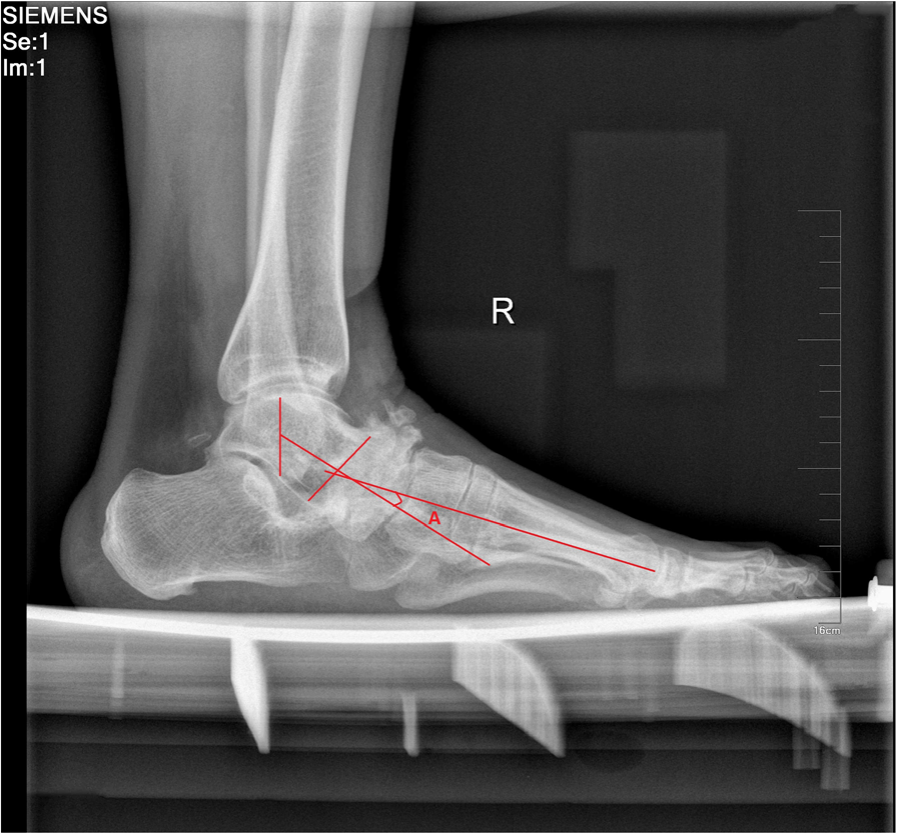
Lateral weightbearing radiograph showing severe sclerosis of the navicular and representation of stage 4 according to the Maceira classification. *Angle A* denotes the Meary-Tomeno’s angle
